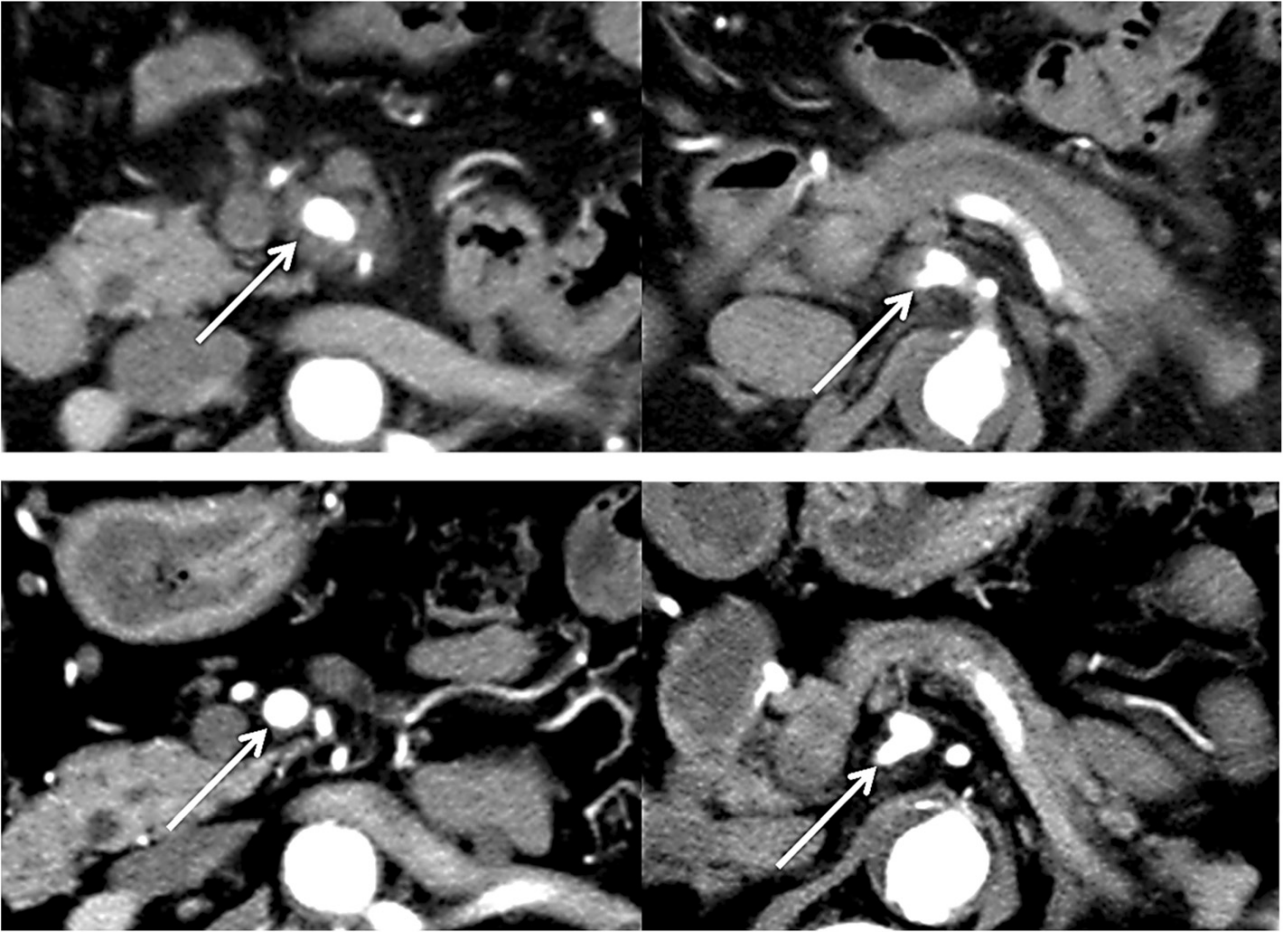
CT scan shows tumor invading to the SMA and CA (*above*). CT scan shows the tumor decreased by chemotherapy (*below*). White arrows indicate tumor invasion to the SMA or CA
